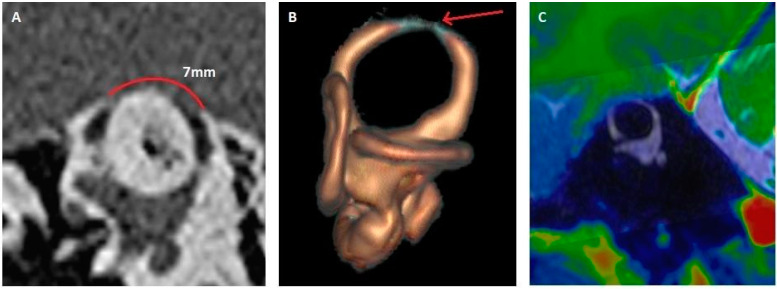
Right SSCD with complete auto-plugging. (A) HRCT, Pöschl plane; dehiscence measured at 7 mm. (B) 3D HR MRI, reconstruction in the plane of the SSC. Complete auto-plugging (arrow) (C) fusion between HRCT and 3D T1 MRI. MRI: magnetic resonance imaging; HRCT: high resolution cranial tomodensitometry; SSCD: superior semicircular canal dehiscence.
